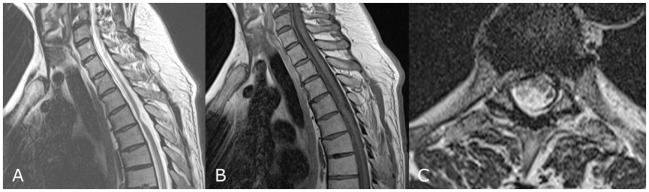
MRI of case 1 showing signs of a herniated spinal cord at T4–T5 at a sagittal T2-weighted image (A), sagittal T1-weighted image (B), and an axial T2-weighted image (C).
